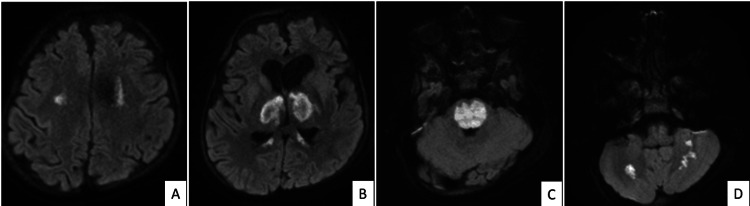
The DWIs (A–D) show bilateral symmetric areas of diffusion restriction in the centrum semiovale (A), thalamus (B), pons (C), and cerebellum (D). DWI: diffusion-weighted image
